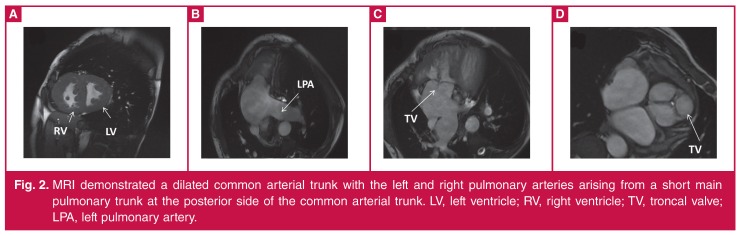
MRI demonstrated a dilated common arterial trunk with the left and right pulmonary arteries arising from a short main pulmonary trunk at the posterior side of the common arterial trunk. LV, left ventricle; RV, right ventricle; TV, troncal valve; LPA, left pulmonary artery.
